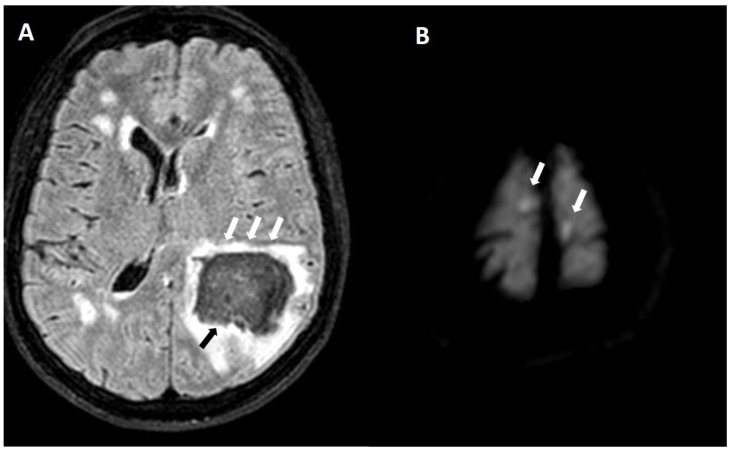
(A) Left parietal lobar hemorrhage (black arrow) with adjacent edema (white arrows) on axial FLAIR (1.5 T, TE = 385 ms, TR = 7000 ms, TI = 2050 ms, slice thickness 3 mm). (B) Bilateral cortical remote diffusion-weighted imaging lesions (arrows) on axial DWI (1.5 T, TE = 57 ms, TR = 2600 ms, b = 1000, slice thickness 5 mm).
